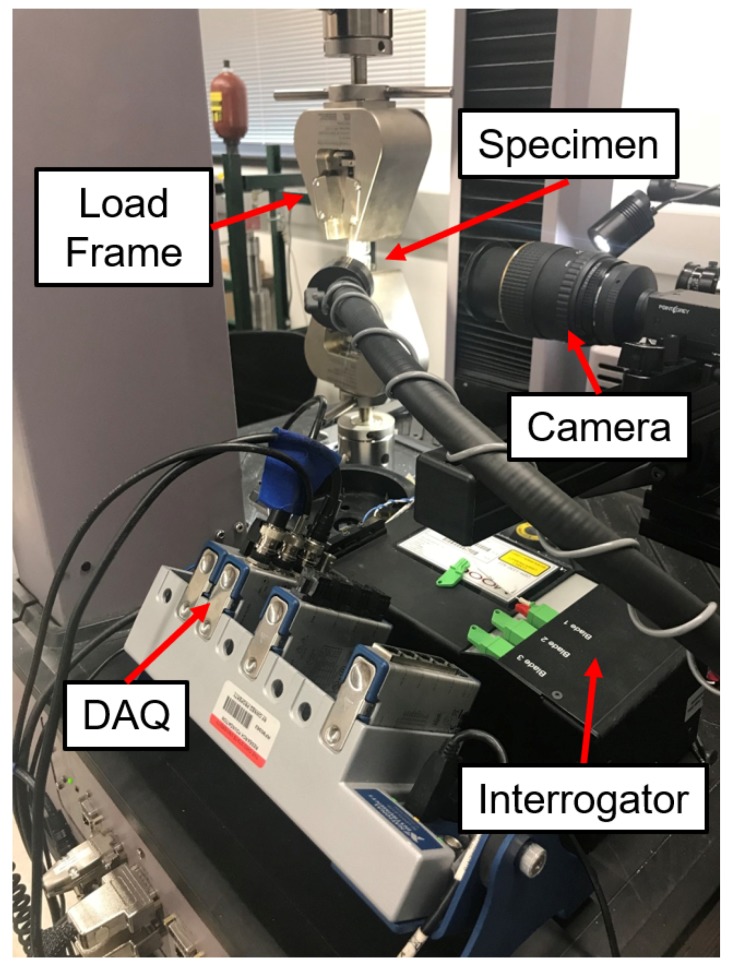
Close-up view of the crack-detection experiment showing a CT coupon installed in the load frame.
